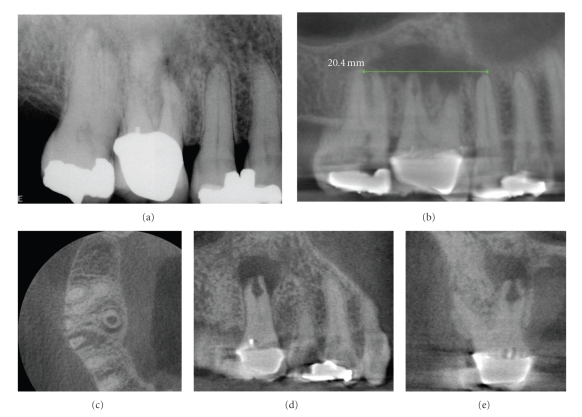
Because two-dimensional imaging suffers from superimposition of anatomic structures, determination of the extent and pathogenesis of periradicular lesions can present diagnostic challenges. This is particularly true of the maxillary posterior region, where the roots of teeth overlap and anatomic structures form complex patterns. A patient presented with discomfort in the maxillary right that extended from the nose to the ear. On clinical examination buccal swelling and induration were present—all teeth tested vital except the maxillary right first molar. A periapical radiograph (a) demonstrated areas of low density at the apices of the maxillary right first and second molars. CBCT images ((b) 10 mm curved planar, (c) axial, (d) sagittal, (e) cross-sectional) however demonstrated a much more extensive (21.4 mm maximum length) unilocular lesion, centered on the palatal root of the maxillary first molar, and extending anteriorly to the second bicuspid and posteriorly to the second molar. Also note the large internal resorptive lesion at the mid-palatal root of the maxillary first molar, not visible on the periapical radiograph. Biopsy confirmed the lesion to be a periapical granuloma with abscess formation.
